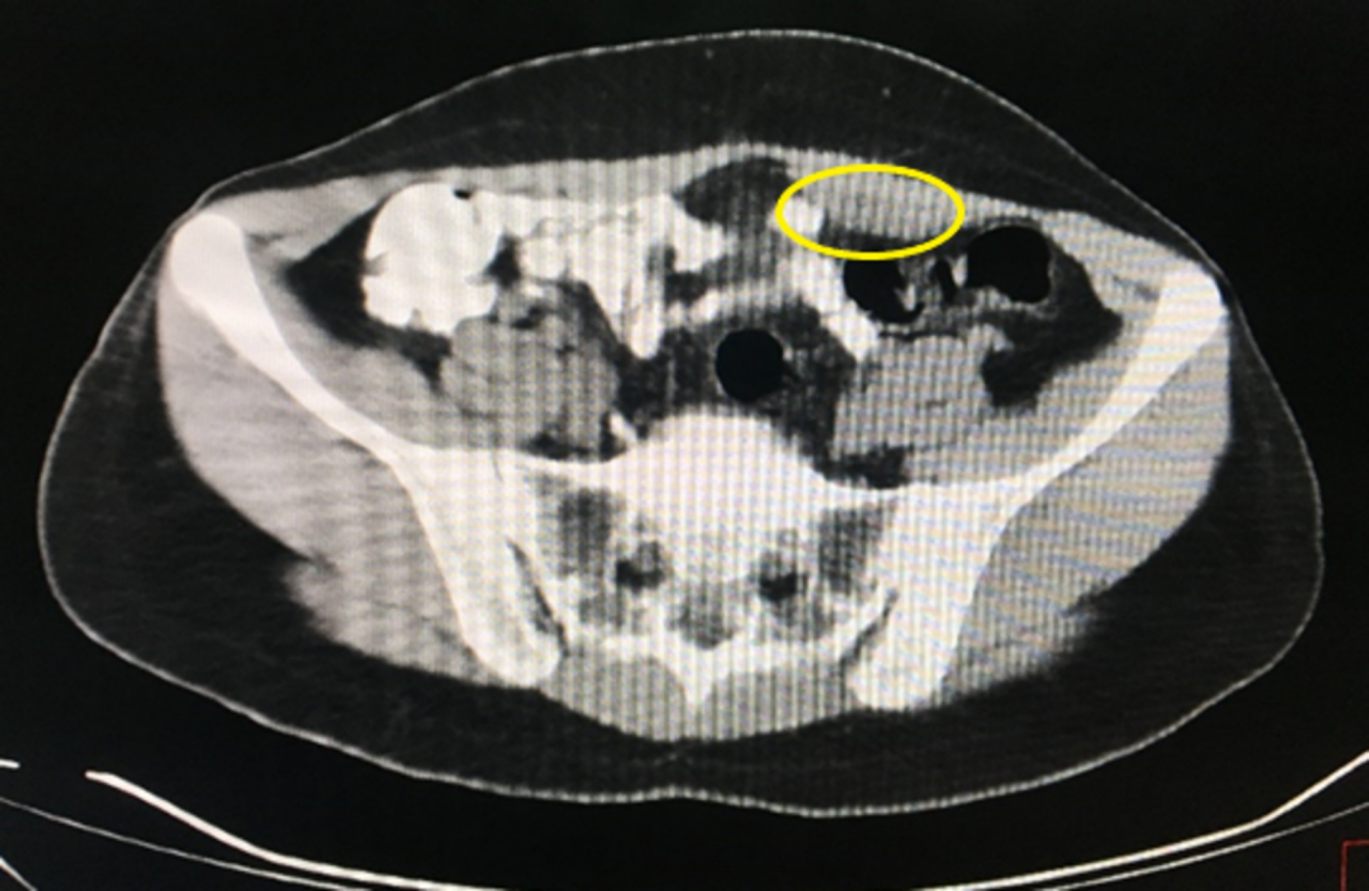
Computed tomography scan showing the endometrioma (yellow circle) contained in the rectus muscle, marked by a hypodensity lesion.
